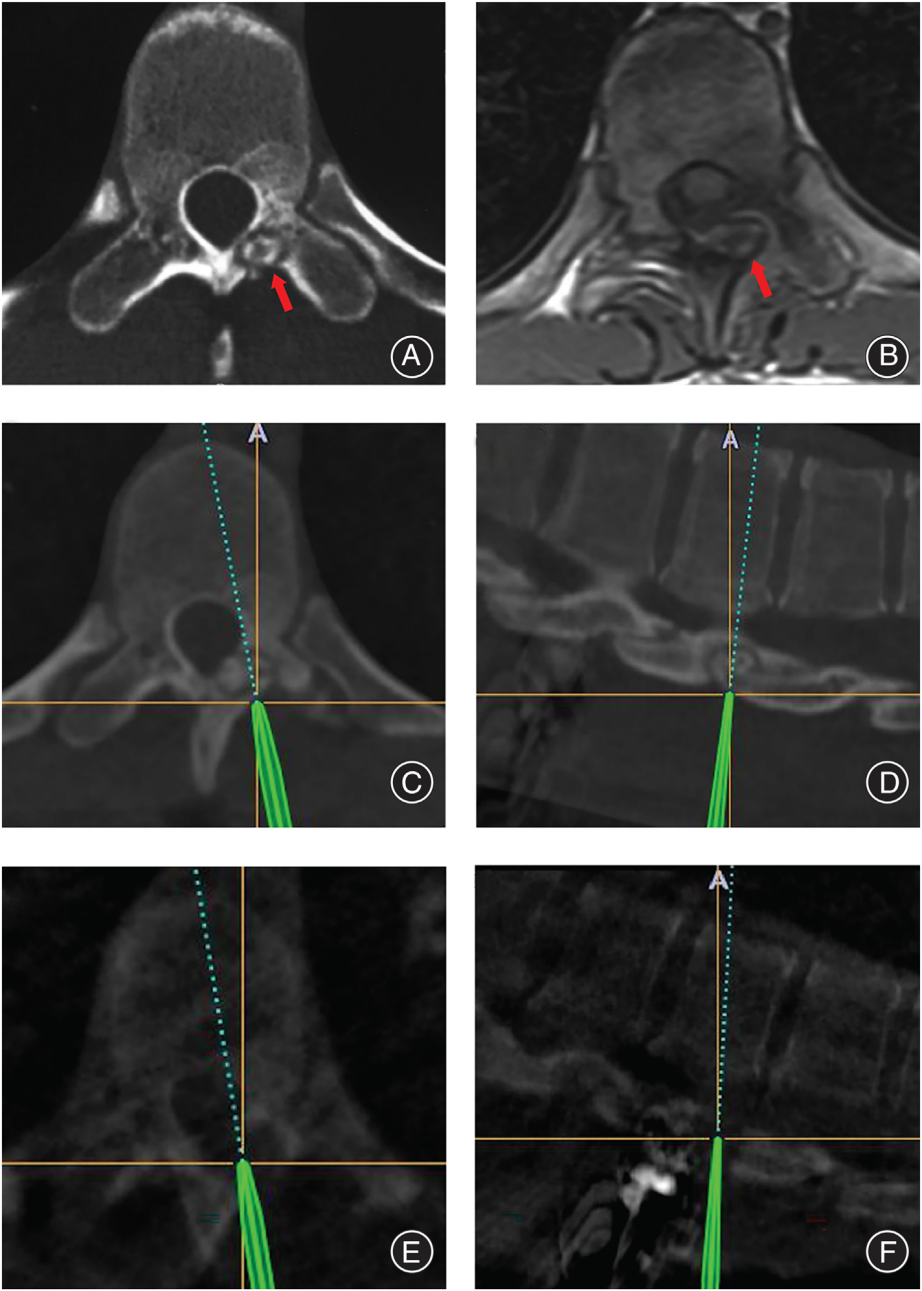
A 30‐year‐old male patient with thoracic spinal osteoid osteoma. (A) CT revealed a well‐defined nidus located in the vertebral laminae of the thoracic spine T_12_. (B) In the same segment of MRI, the signal of the nidus was obscure with no strong contrast with surrounding bone. (C, D) Computer‐assisted navigation was used in the surgery, to obtain precise location of the nidus simultaneously in three‐dimensions. (E, F) After curettage of the nidus, intraoperative CT was performed to confirm the tumor was completely removed.
